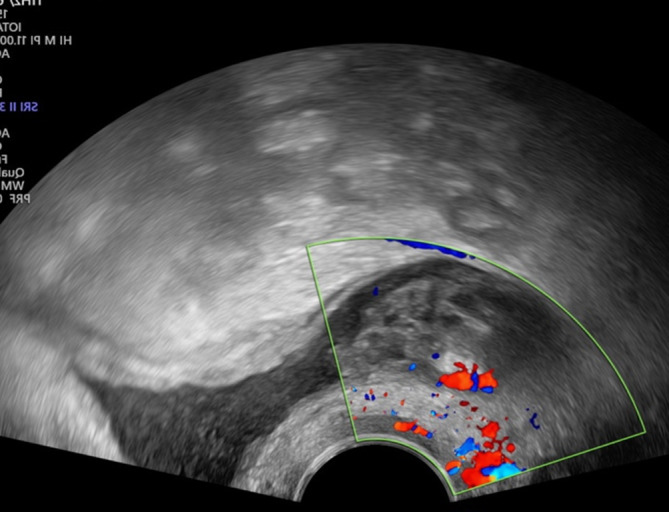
Bei der Perinealsonographie erfolgt die externe sagittale Applikation einer Abdominalsonde im Bereich des Introitus vaginae mit einem möglichst geringen Auflagedruck, um einer Kompression des darunter gelegenen Gewebes vorzubeugen [12, 15]. Die Perinealsonographie ermöglicht insbesondere die Darstellung von Symphyse, Blase und Urethra, sodass die Blasenhalsregion mit ihren funktionellen Veränderungen beurteilt werden kann [6].
